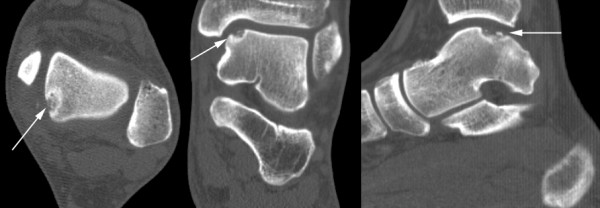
Preoperative computed tomography (axial, coronal, and sagittal slices) of the left ankle of a 25-year-old female showing a typical osteochondral defect located on the posteromedial talar dome (arrows).
